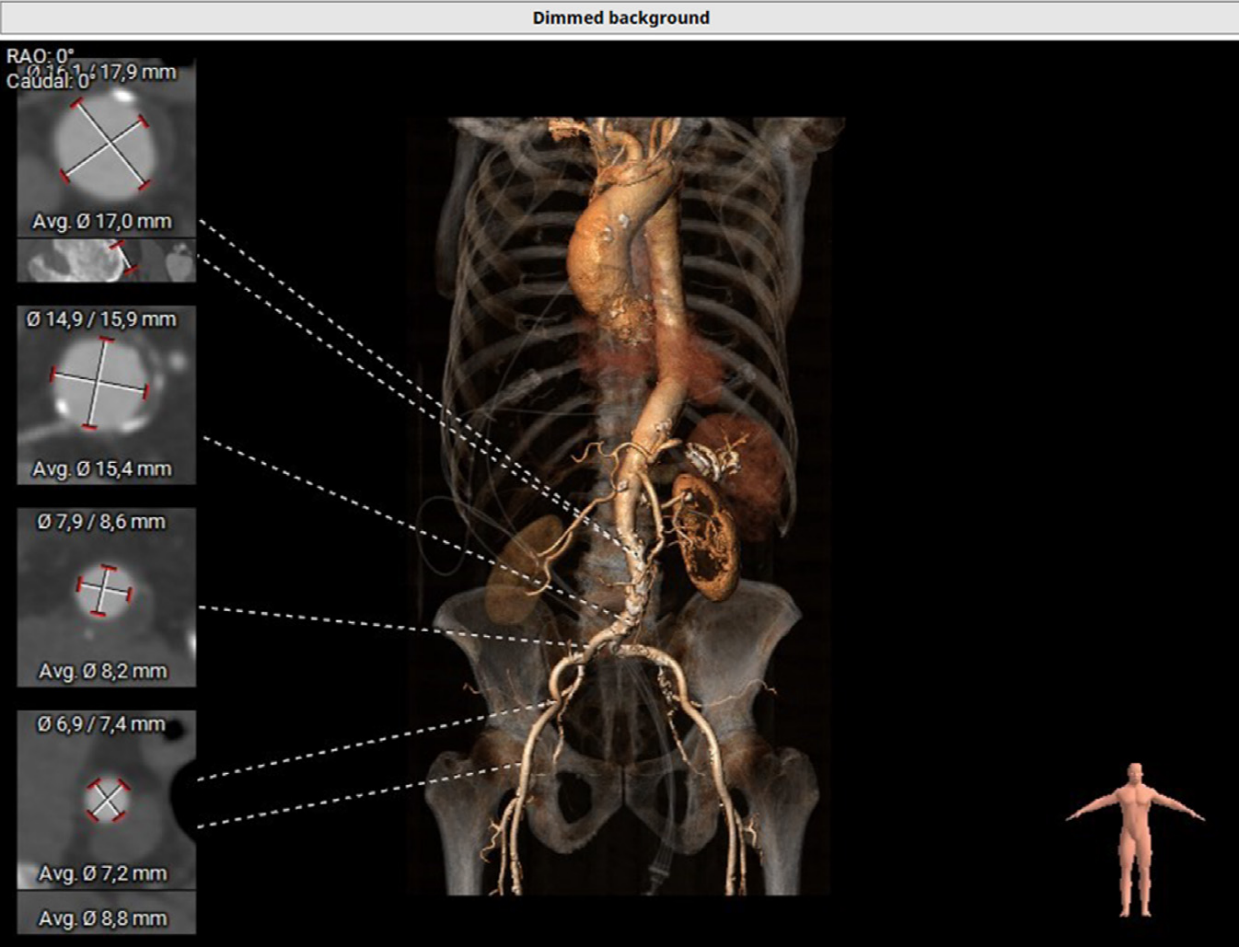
Peripheral Accesses on Preoperative CT Three-dimensional reconstruction of the left and right iliac and femoral arteries, with short-axis measurements. CT = computed tomography; RAO = right anterior oblique.
